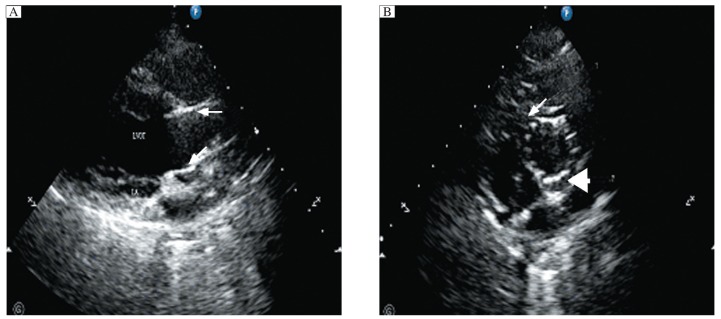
Echocardiographic image of a 23-mm device implanted into an aortic root with a 19.1-mm aortic annulus (2 h post procedure). Echocardiography showed that the device was in a good position and functioned normally. A: Long-axis, the stent (arrows) showed strong echo signals (LA=left atrium, LVOT=left ventricular outflow tract). B: Short-axis, the right coronary sinus (arrow) and left coronary sinus (arrowhead) were unaffected.
